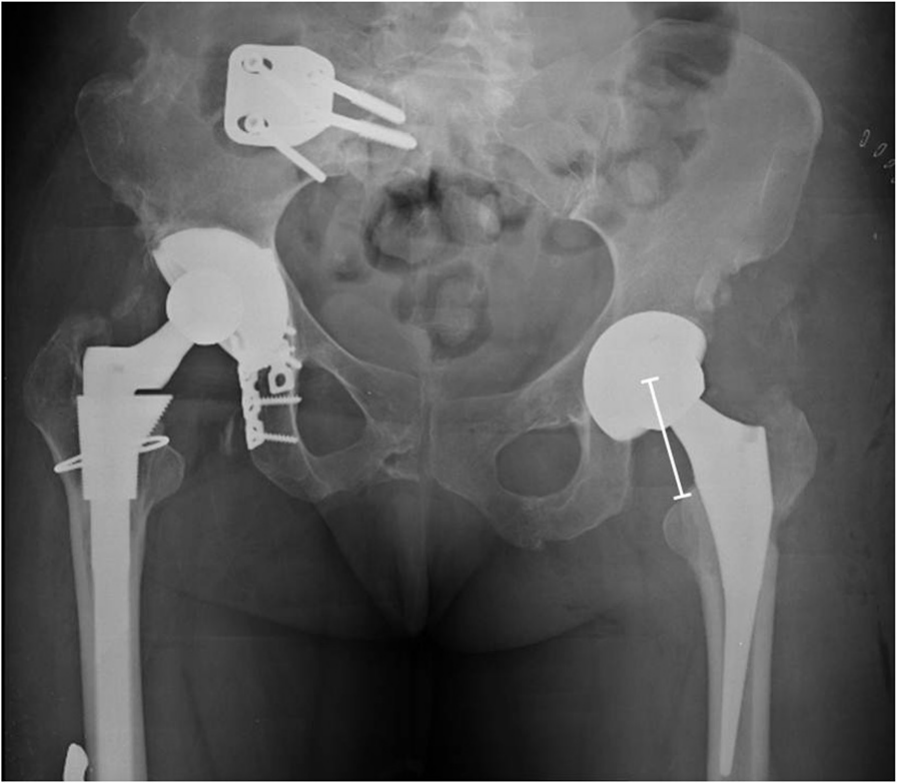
Anteroposterior pelvic radiograph after the revision left total hip arthroplasty. Note the decreased distance between femoral head center of rotation to the lesser trochanter, compared to Figure 5.
